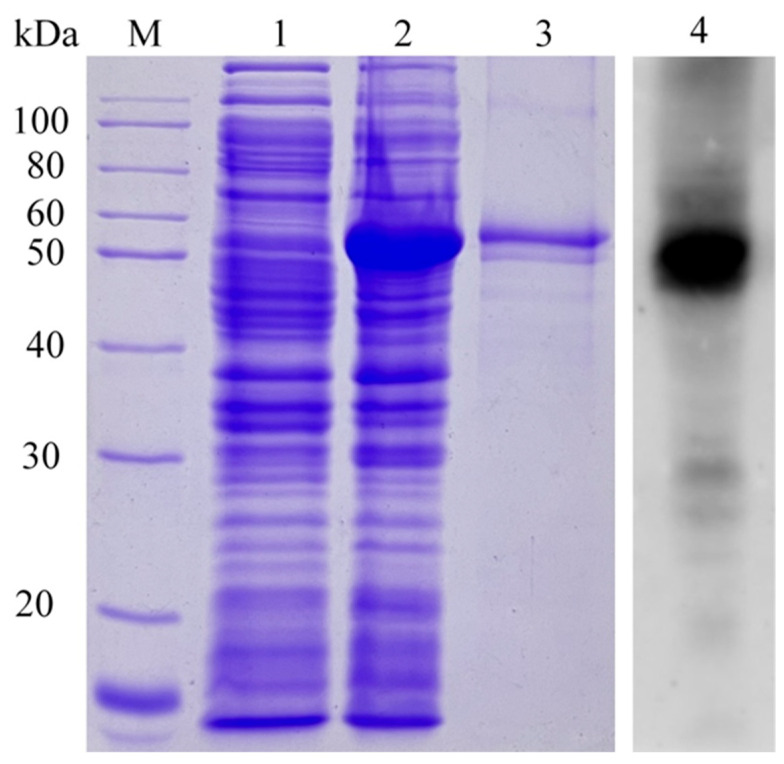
Analysis of RuXyn by SDS-PAGE and Western blotting. M, protein marker; 1, nontransformed *E. coli* BL21(DE3); 2, RuXyn transformants induced with 0.2 mM IPTG; 3, purified RuXyn; 4, Western blot analysis of supernatant from the ultrasonication of RuXyn transformants.
